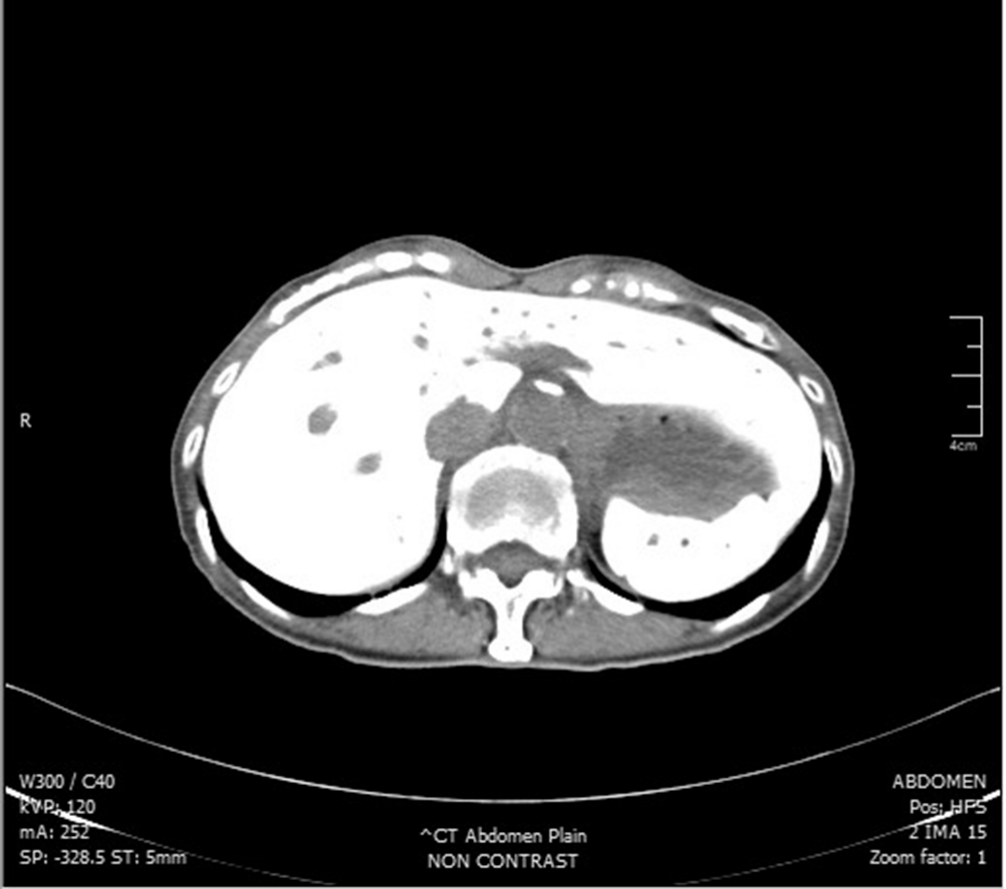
Plain CT abdomen showing barium within the liver and spleen.
